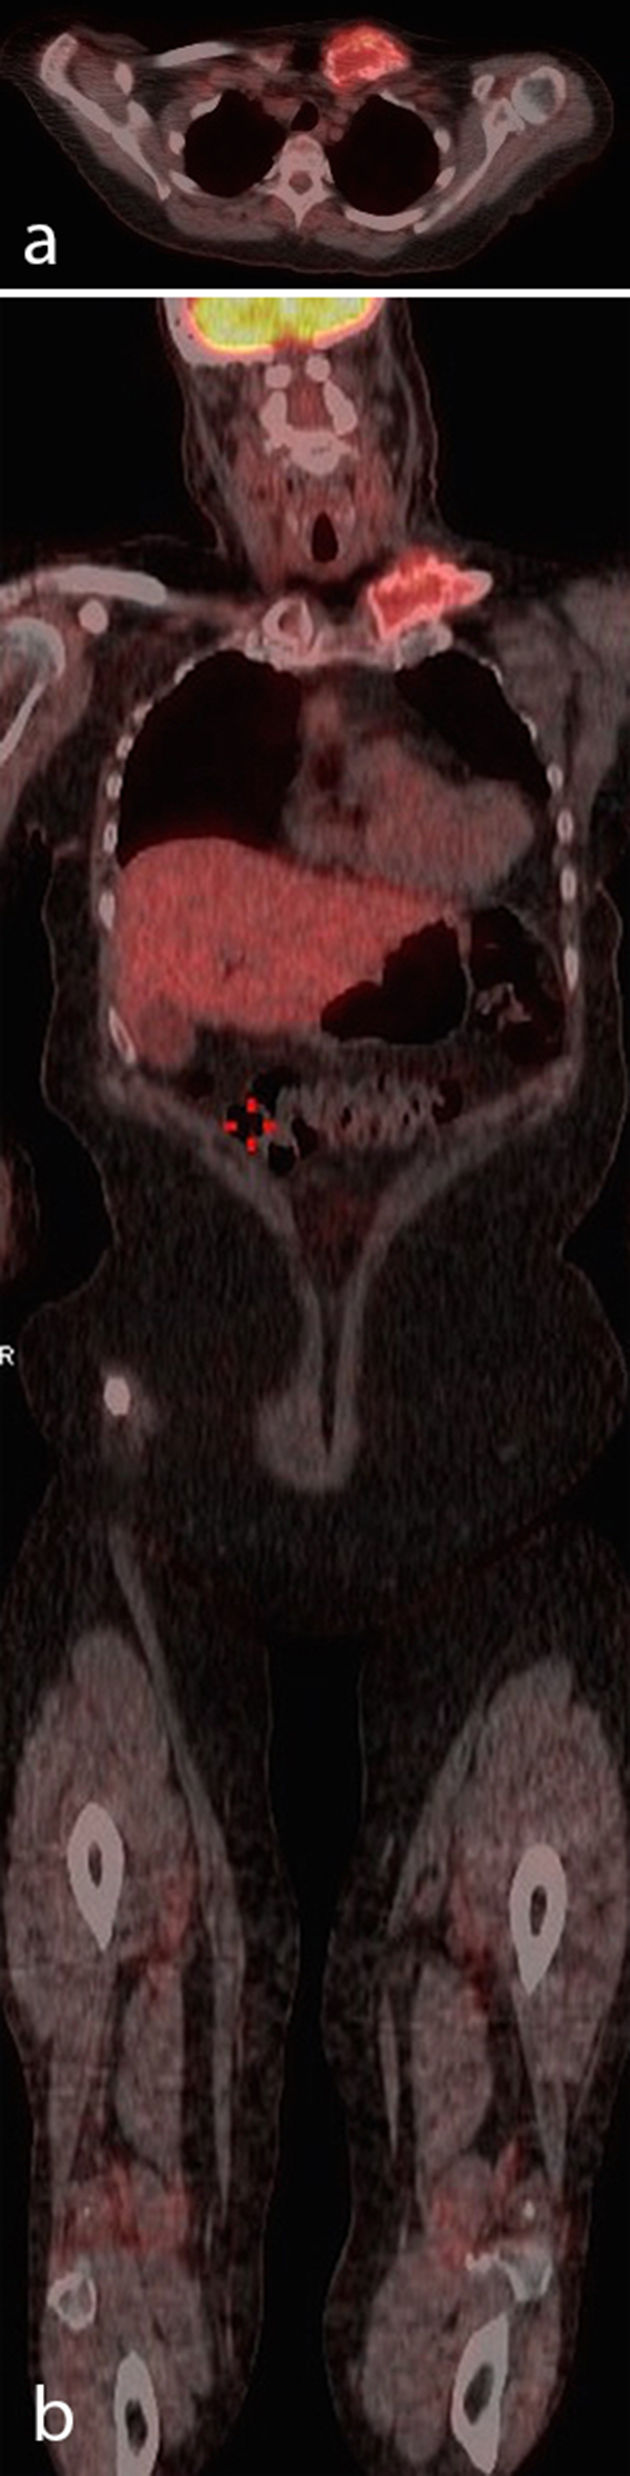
(a) Axial FDG-PET/CT slice showing bony destruction of the medial end of the clavicle with associated soft tissue and demonstrating moderate tracer uptake (SUV_max_ = 4.7). (b) Coronal FDG-PET/CT slice showing the medial left clavicle lesion. FDG-PET, fludeoxyglucose-positron emission tomography; SUV, standardized uptake value.
